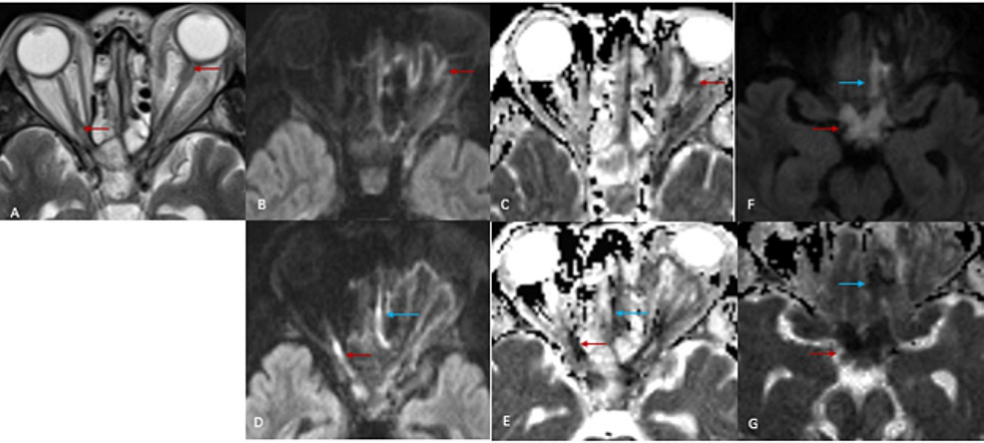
Intra-orbital and optic pathway involvement continued (A)-(E): Bilateral optic nerve involvement: (A) T2WI for anatomy; red arrows correspond to diffusion restriction on (B) to (E);  (B)-(E) DWI and ADC: diffusion restriction of left and right optic nerves (red arrows). Also noted diffusion restriction in the left olfactory tract (blue arrows); (F)-(G): DWI and ADC: diffusion restriction in the optic chiasm (red arrows) and left gyrus rectus along the olfactory tract (blue arrows) DWI: diffusion weighted imaging; ADC: apparent diffusion coefficient; T2WI: T2 weighted image
